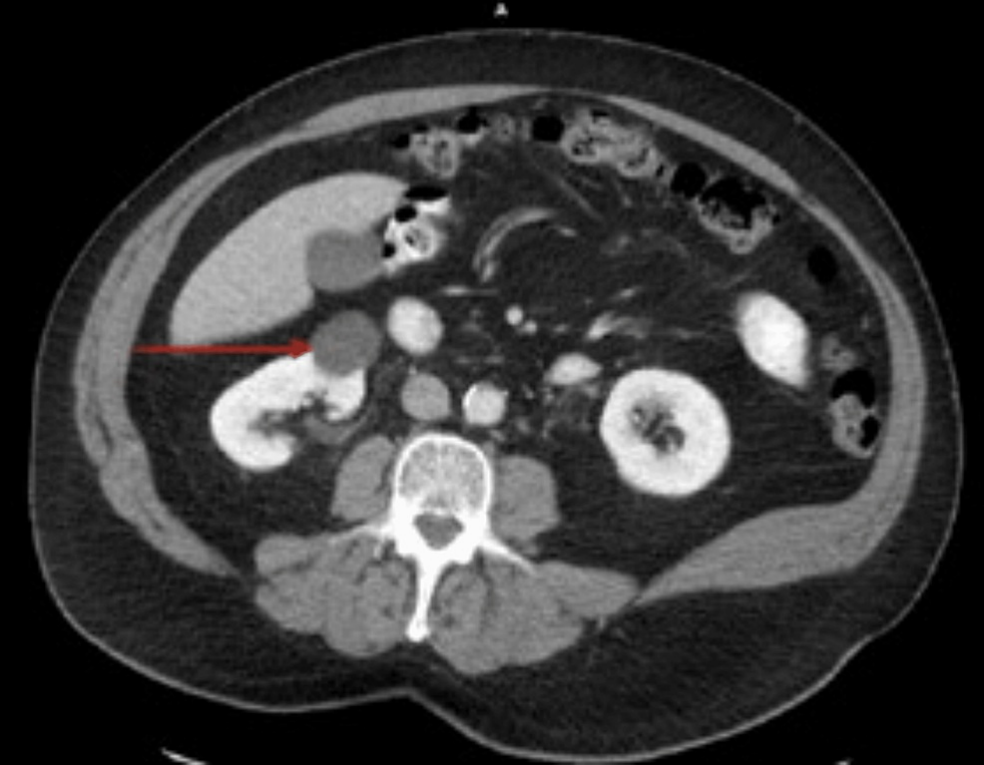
MRI lumbar region showing nodules (red arrow)
